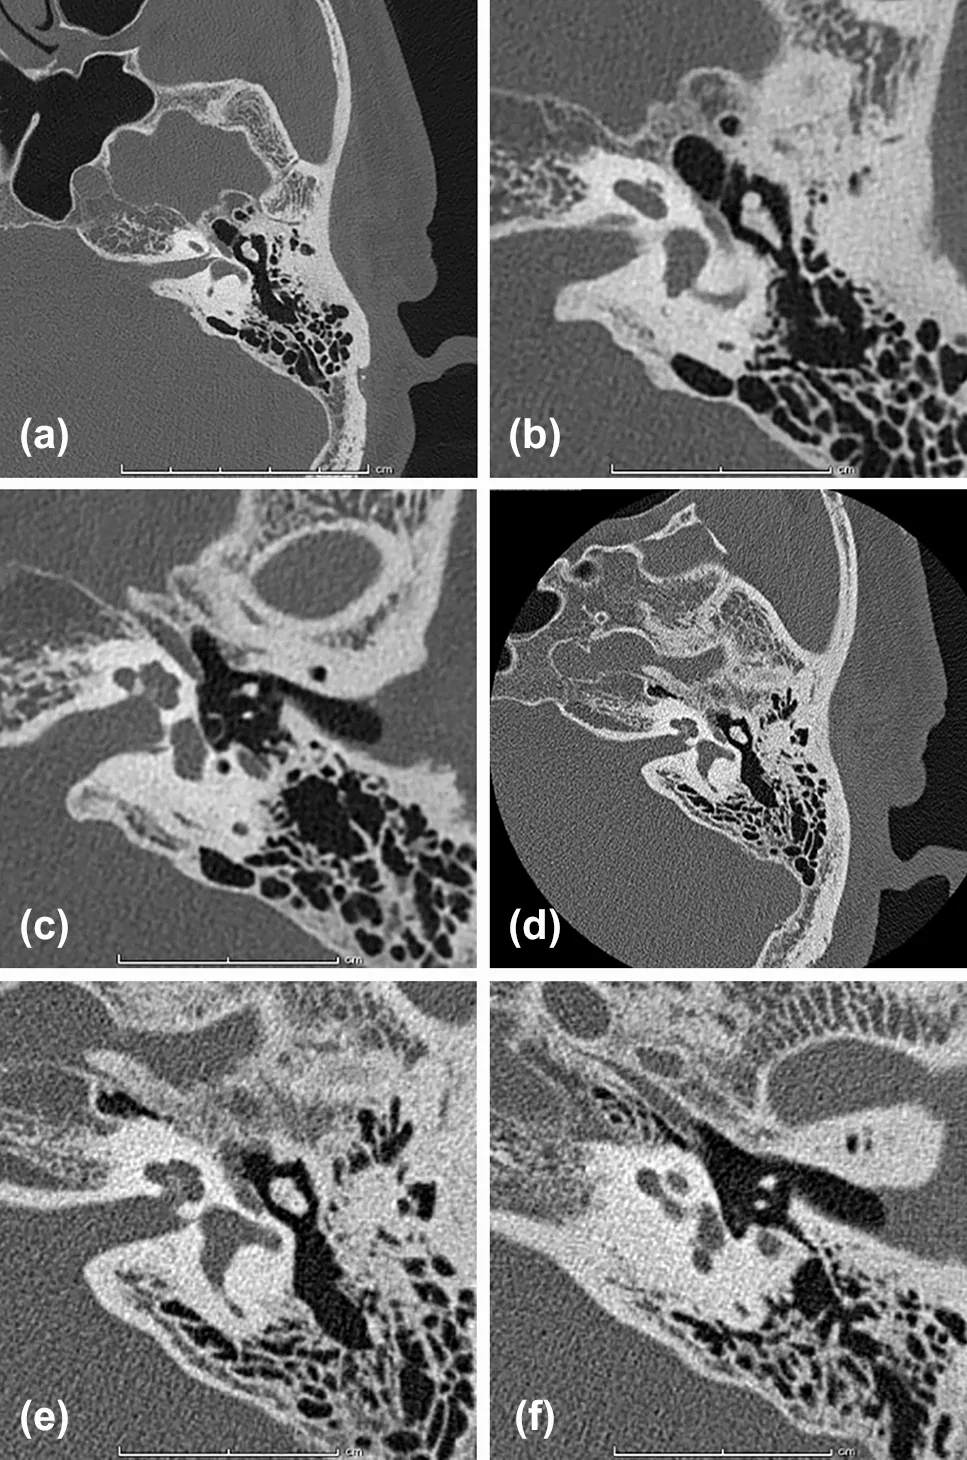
PCD-CT axial images of the left temporal bone CT at standard dose (a–c). The visual scores for both the incudomalleolar joint (b) and stapes (c) by two reviewers were 4. EID-CT axial images of the left temporal bone CT at standard dose (d–f). The visual scores for the incudomalleolar joint (e) and stapes (f) by two reviewers were both 2 PCD-CT, photon-counting detector computed tomography; EID-CT, energy-integrating detector computed tomography
